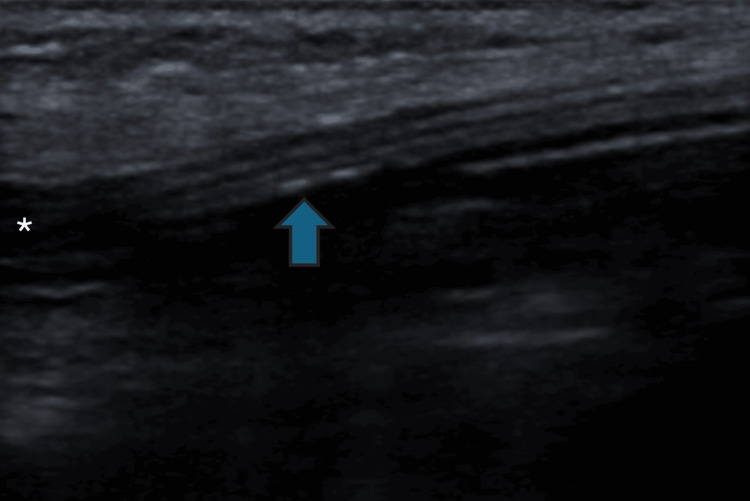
Ultrasound of right external jugular vein. The ultrasound shows the tip of the central venous catheter (blue arrow) inside the right external jugular vein (*).
